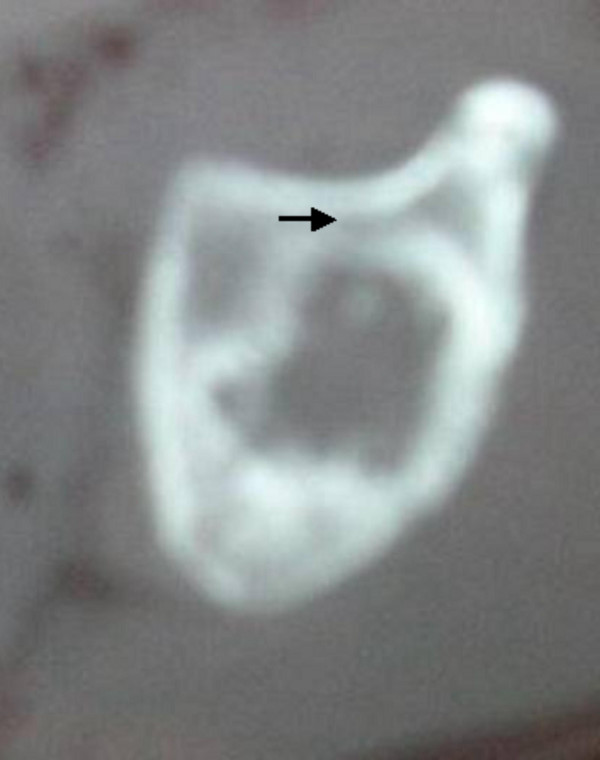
Patient 1: Axial CT scan at age of 17 years shows a necrotic acetabular roof (arrow).
